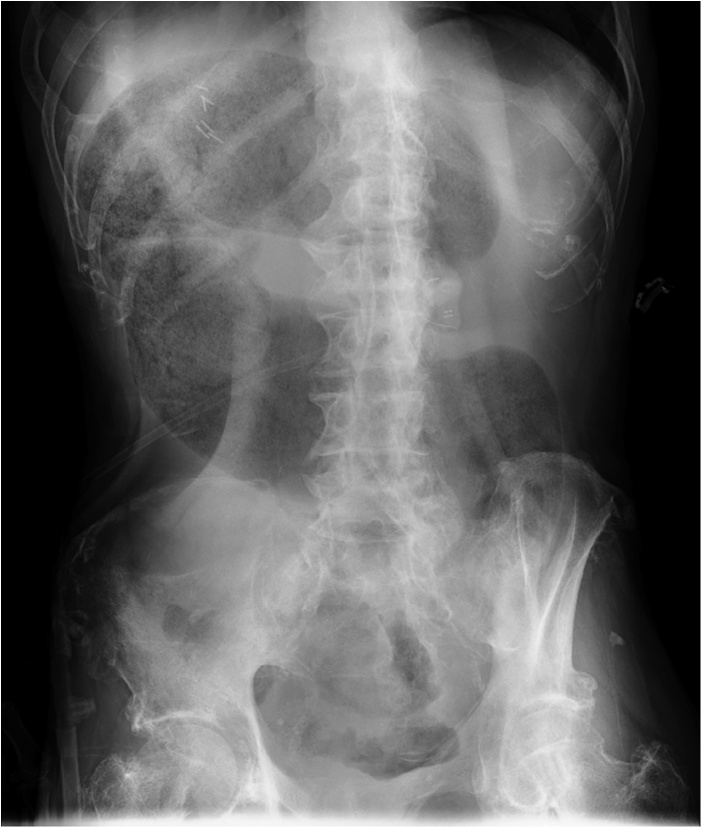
Distended sigmoid colon showing "coffee bean sign" on the abdominal X-ray.
